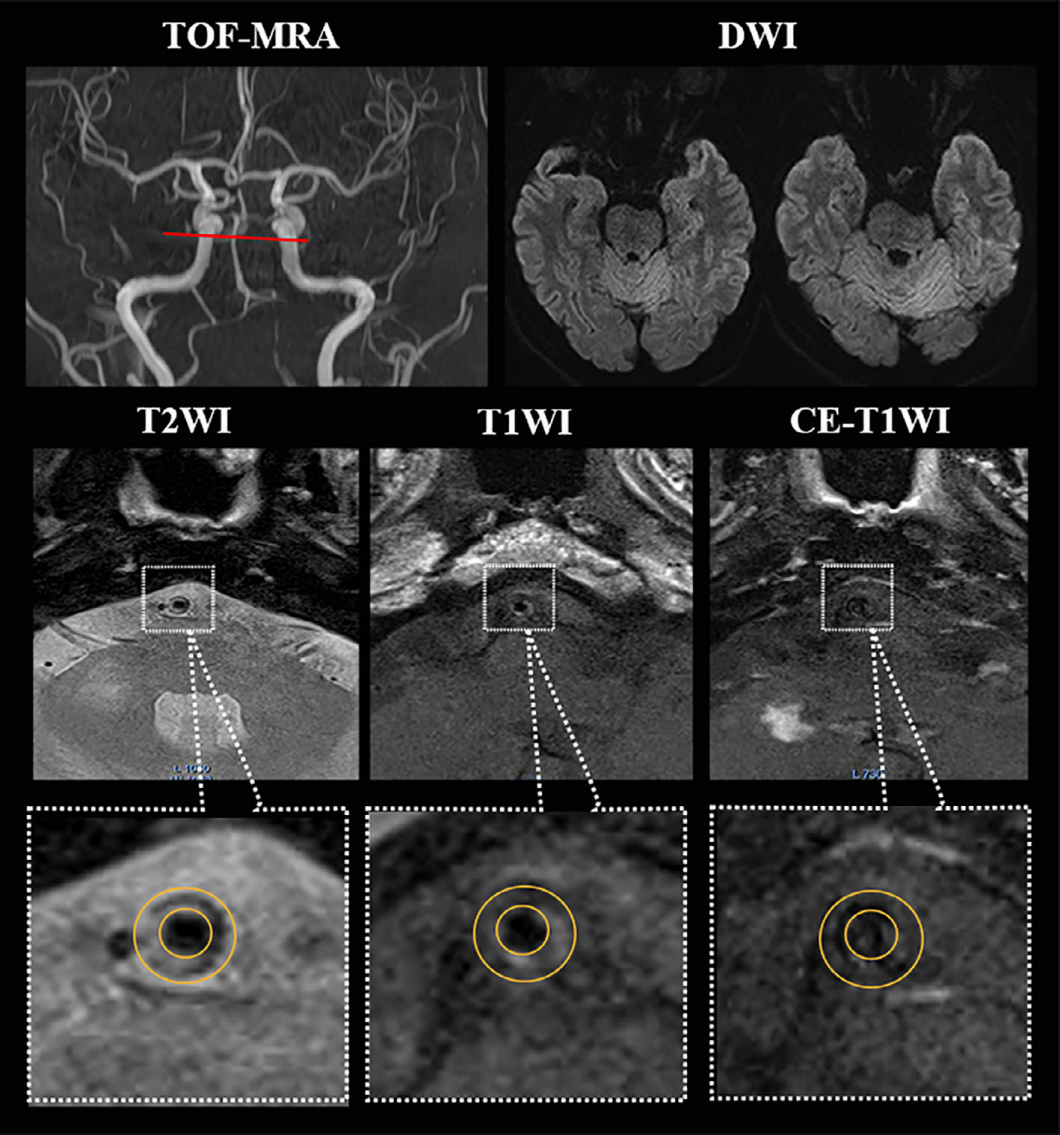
hrMRI images showing intracranial atherosclerotic plaque on basilar artery. A 56‐year‐old woman without hypertension having acute ischemic symptom with 2 weeks; TOF‐MRA showing a <50% stenosis of BA (red line on the most stenotic site) and DWI without any fresh infarct; hrMRI images showing an eccentric plaque with the nonstenotic lumen (small yellow circle); the hrMRI parameters (minimal luminal area = 2.71 mm^2^; plaque volume = 14.02 mm^3^; plaque burden = 78.8%; remodeling ratio = 71.1%; eccentricity index = 0.63; enhancement ratio = 29.5%) were obtained; after standard medical management, she had achieved 0 score of the mRS at day 90.
